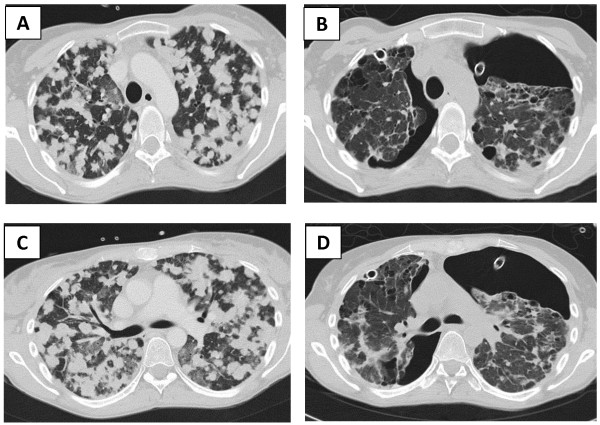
Thoracic computed tomography scan. (A) (C) Initial thoracic computed tomography scan showing multiple pulmonary metastases. (B) (D) Thoracic computed tomography scan after 2 months of treatment with erlotinib, showing a partial thoracic response with the appearance of pulmonary cysts and a partially drained bilateral pneumothorax.
